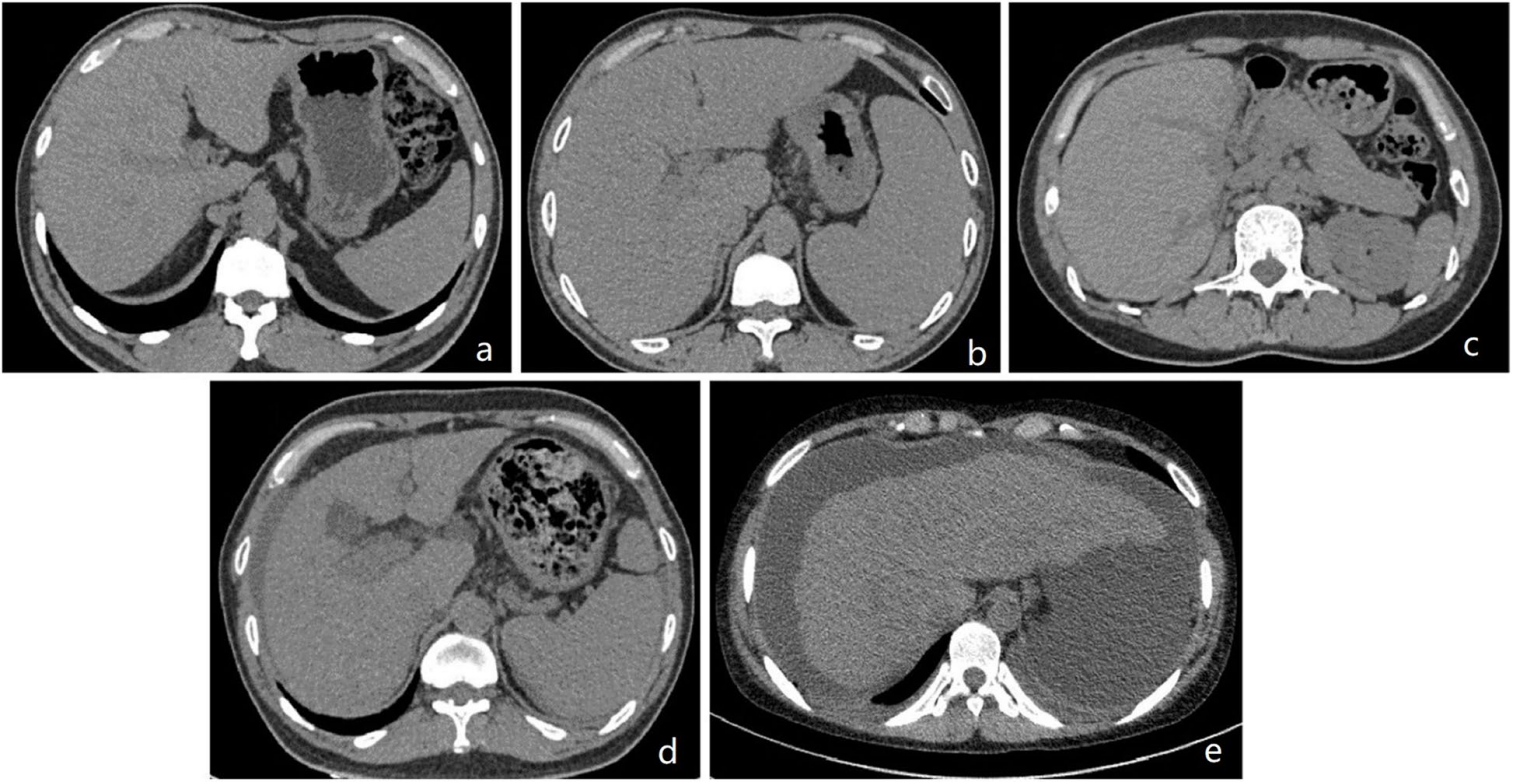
Computed tomography findings of liver fibrosis at each stage. a Fibrosis grade 0 (F0): normal liver. b Fibrosis grade 1 (F1): no significant change in liver volume and increased volume of spleen. c Fibrosis grade 2 (F2): the liver volume is slightly reduced. d Fibrosis grade 3 (F3): portal vein thickening, spleen enlargement, and minimal ascites are visible. e Fibrosis grade 4 (F4): liver with an irregular shape and ascites are visible
